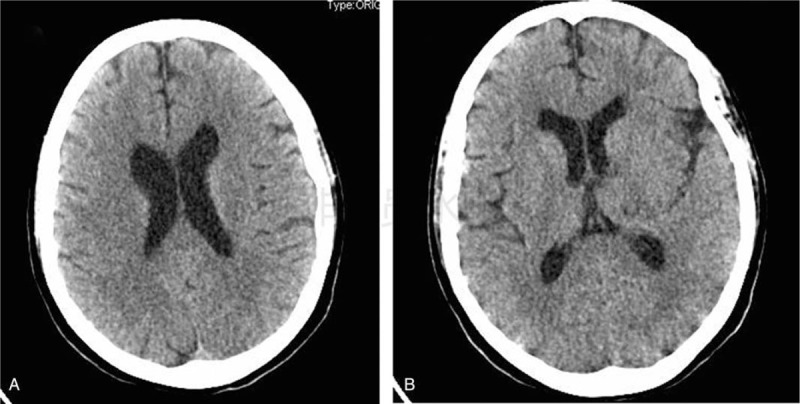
The computed tomography imaging at 1-year post-discharge (A, B) shows near normal brain findings.
